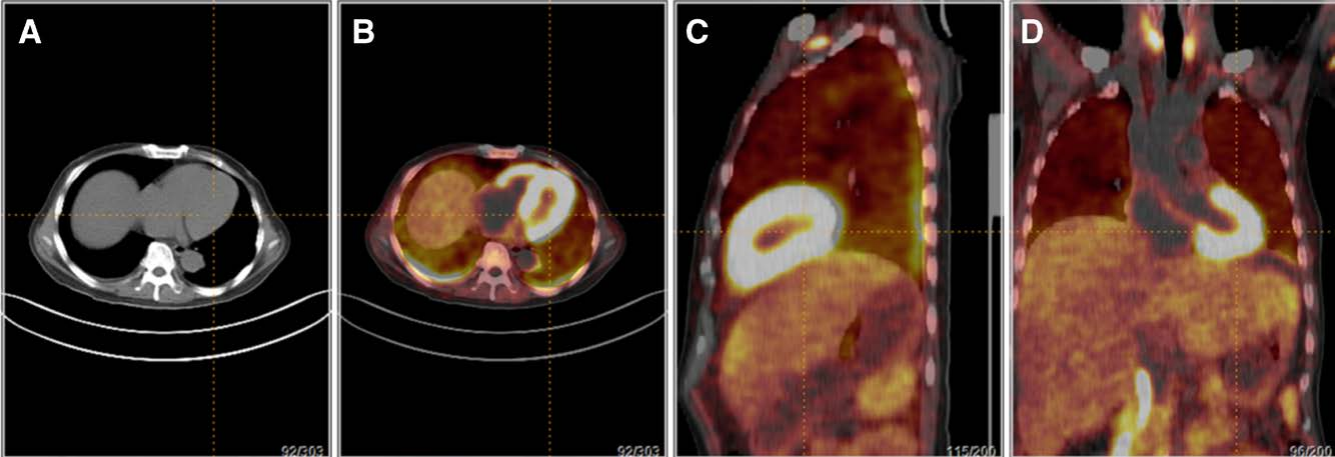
18F-AV45 PET-CT: unevenly increased radiotracer uptake is observed in the heart, liver, spleen, omentum, mesentery, pelvic peritoneum, and bone marrow.
